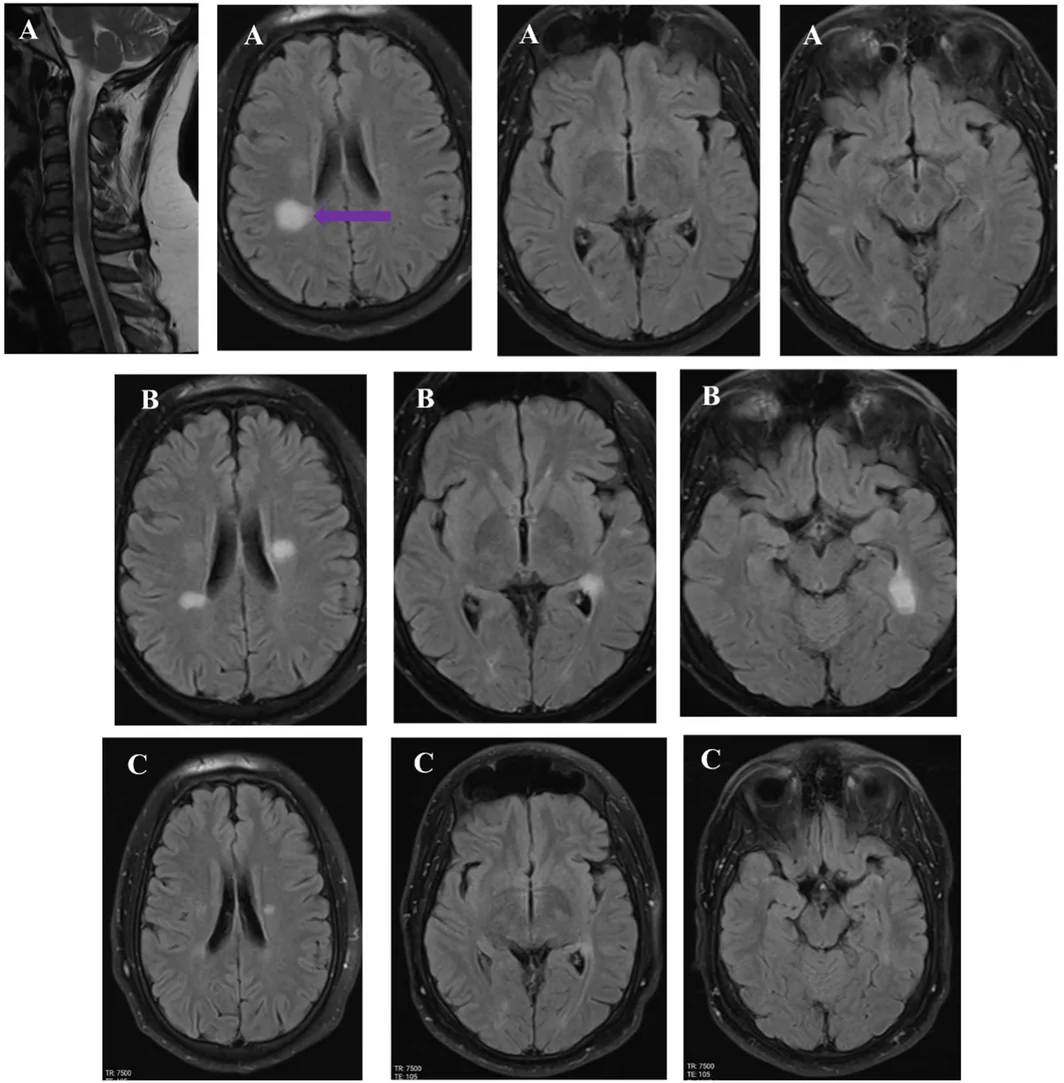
Patient's MRIs: (A) first MRI shows a short‐segment lesion in the cervical cord and periventricular lesions, one of them was TDL marked with an arrow; (B) second MRI after exacerbation of symptoms shows improvement in prior lesions, and the emergence of two new periventricular lesions, one of them was TDL marked with an arrow; and (C) shows a significant reduction in lesion size after 6 months of treatment.
